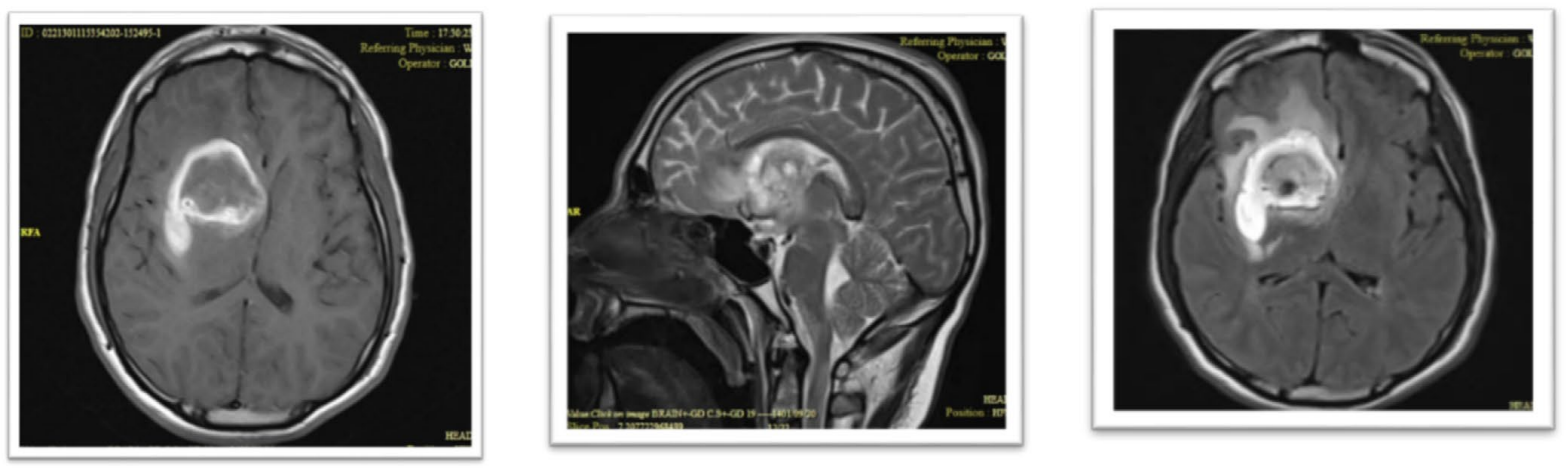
(Left) Axial T1‐weighted, (Middle) T2‐weighted, and (Right) FLAIR images demonstrate a heterogeneous signal focus in the right frontoparietal region consistent with acute hemorrhage, with extensive surrounding vasogenic edema.
